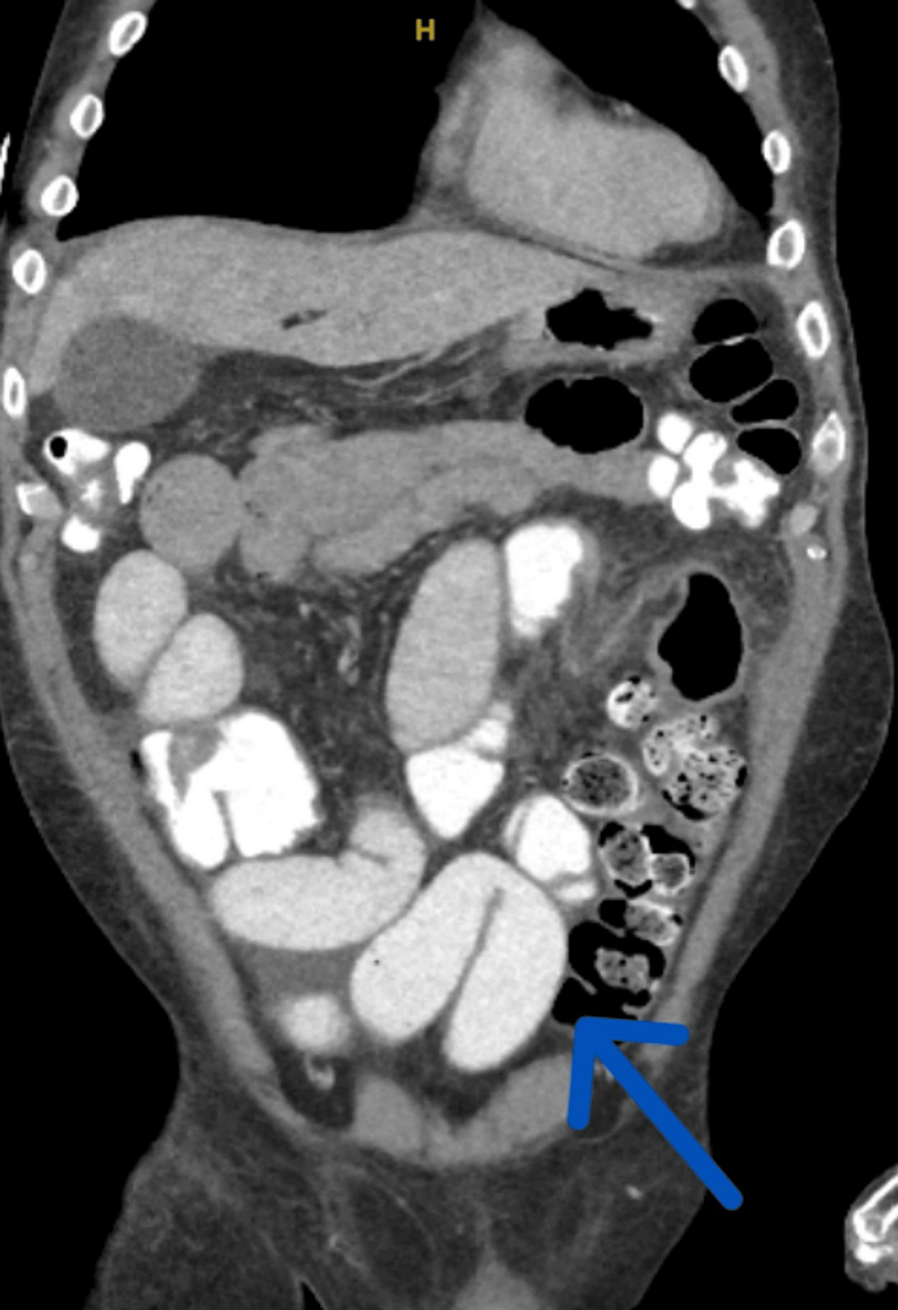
CT scan of the abdomen showing possible ileitis
